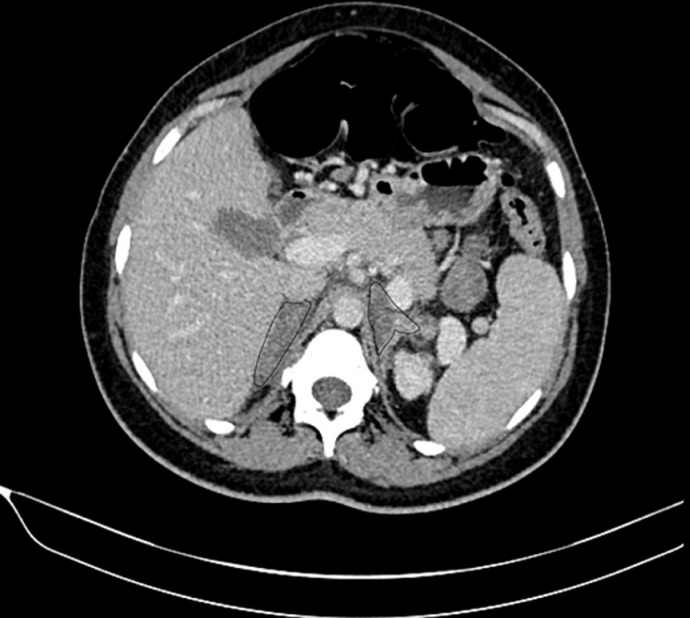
axial view of contrast-enhanced abdominopelvic computed tomography showing bilateral adrenal enlargement with lack of contrast enhancement in a 34-week pregnant patient with bilateral adrenal infarction
